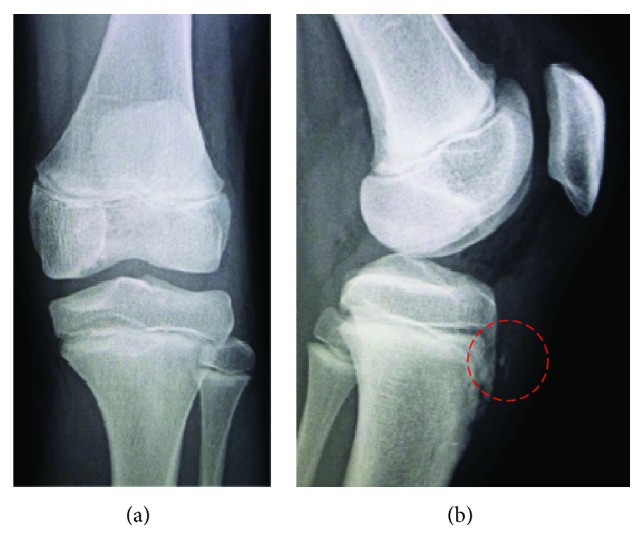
X-rays and computed tomography (CT) showed small flecks of bone at proximal tibial tuberosity.
